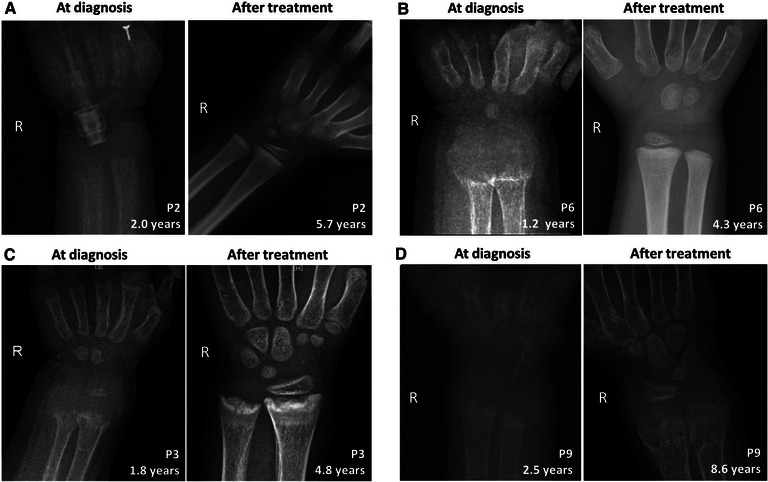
Comparison of skeletal x-ray images of four VDDR1A children at diagnosis and after treatment. In each panel, the left is the skeletal x-ray image at diagnosis, while the right is the image after treatment. P2 and P6 showed good compliance, while P3 and P9 showed poor compliance. (A) P2, (B) P6, (C) P3, and (D) P9.
